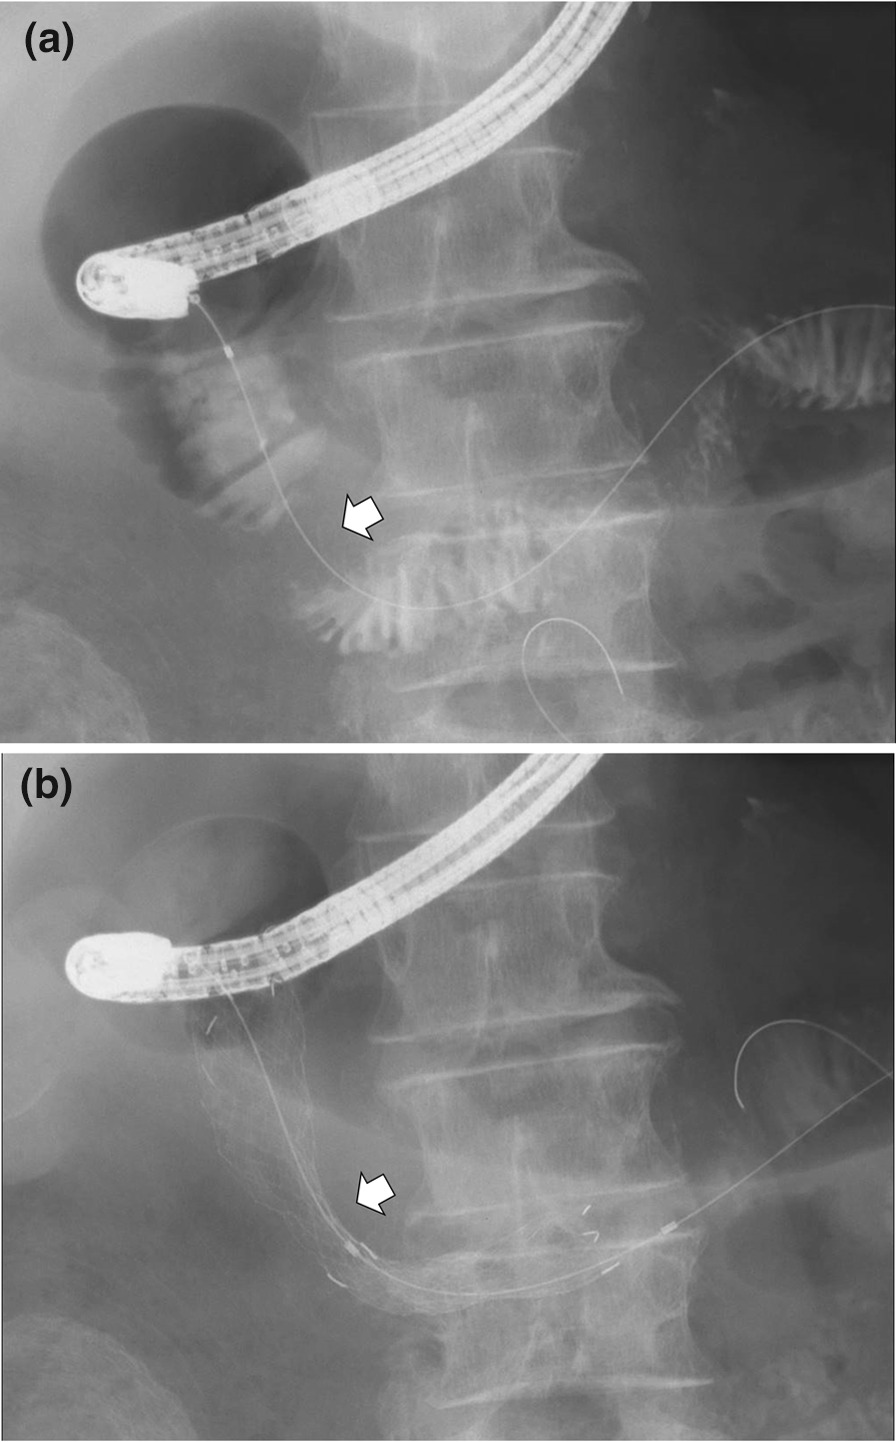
The process of duodenal stent placement. a A catheter with a guidewire is passed through the stenosis site (white arrow), and a water-soluble radiographic contrast medium is injected to determine the location and length of the stenosis site under fluoroscopic guidance. b A duodenal stent is deployed under endoscopic and fluoroscopic guidance. White arrow is showing stenosis cite
